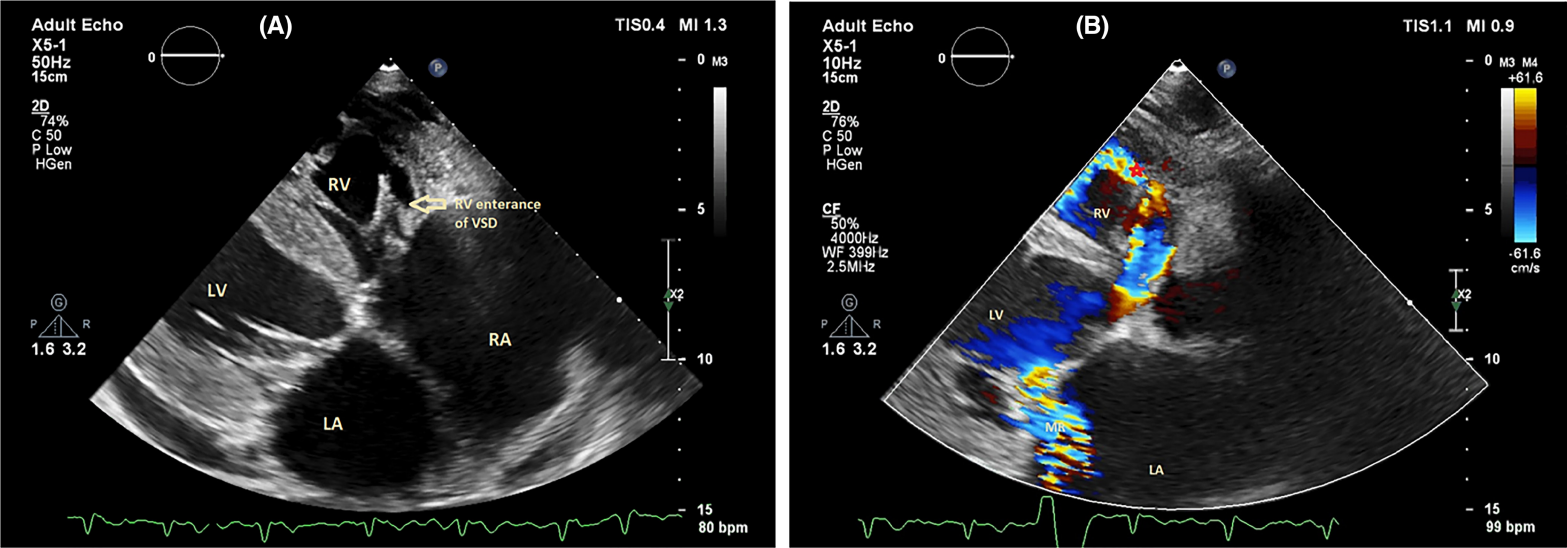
Transthoracic echocardiography in the off‐axis view shows the RV entrance site of VSD tunnel (A), Color Doppler image shows a turbulent flow of VSD. LA, left atrium; LV, left ventricle; MR, mitral regurgitation; RA, right atrium; RV, right ventricle; VSD, ventricular septal defect.
